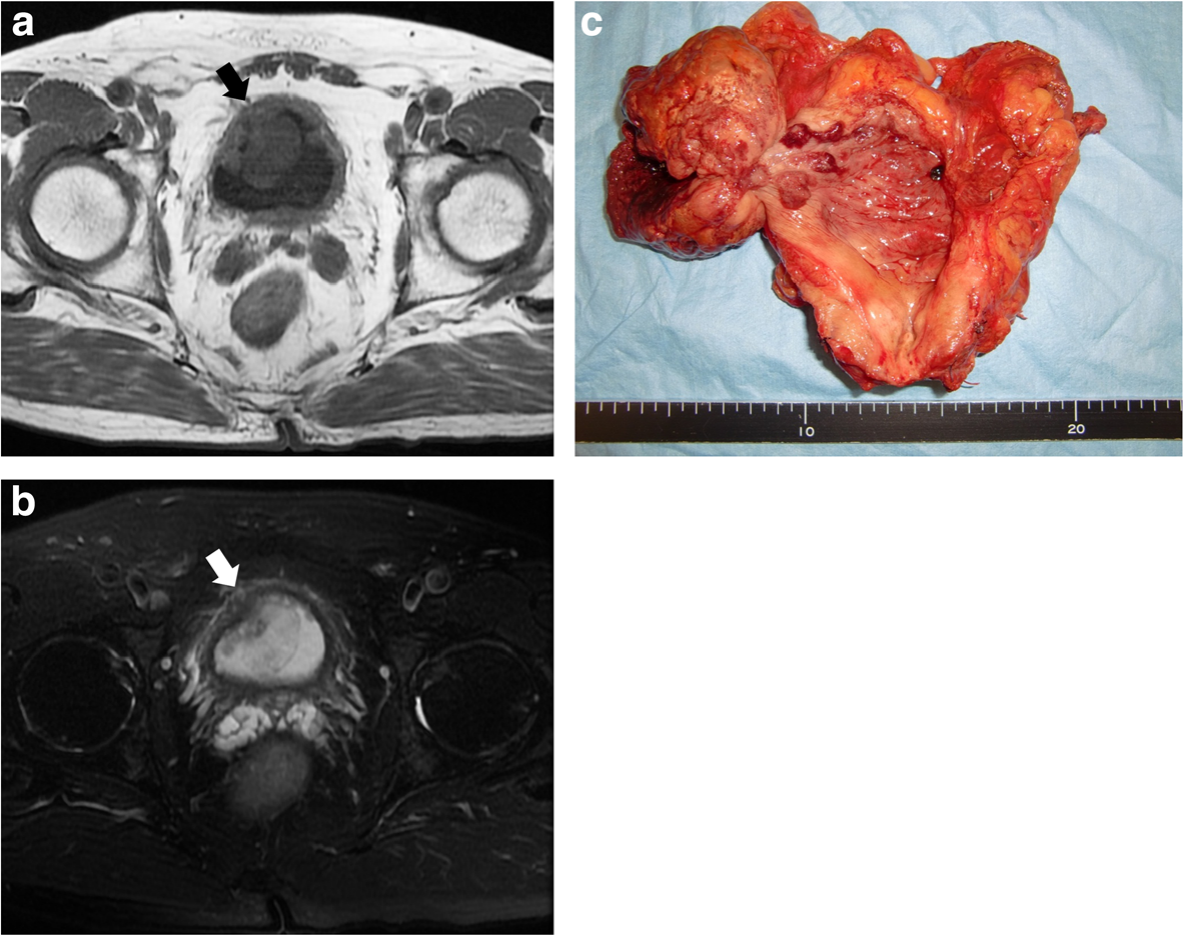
Magnetic resonance imaging scans obtained at presentation (a and b) and of the resected urinary bladder (c). Magnetic resonance imaging demonstrated a large mass (3.8cm×2.9cm) with fragmentation of muscularis propria (*arrows*), which was located in the anterior wall of the urinary bladder. The mass intensity was the same as in the rest of the bladder wall on T1-weighted images (a) and slightly greater than in the bladder wall on T2-weighted ones (b). The resected urinary bladder showed a large pedunculated polyp with a surrounding papillary appearance
